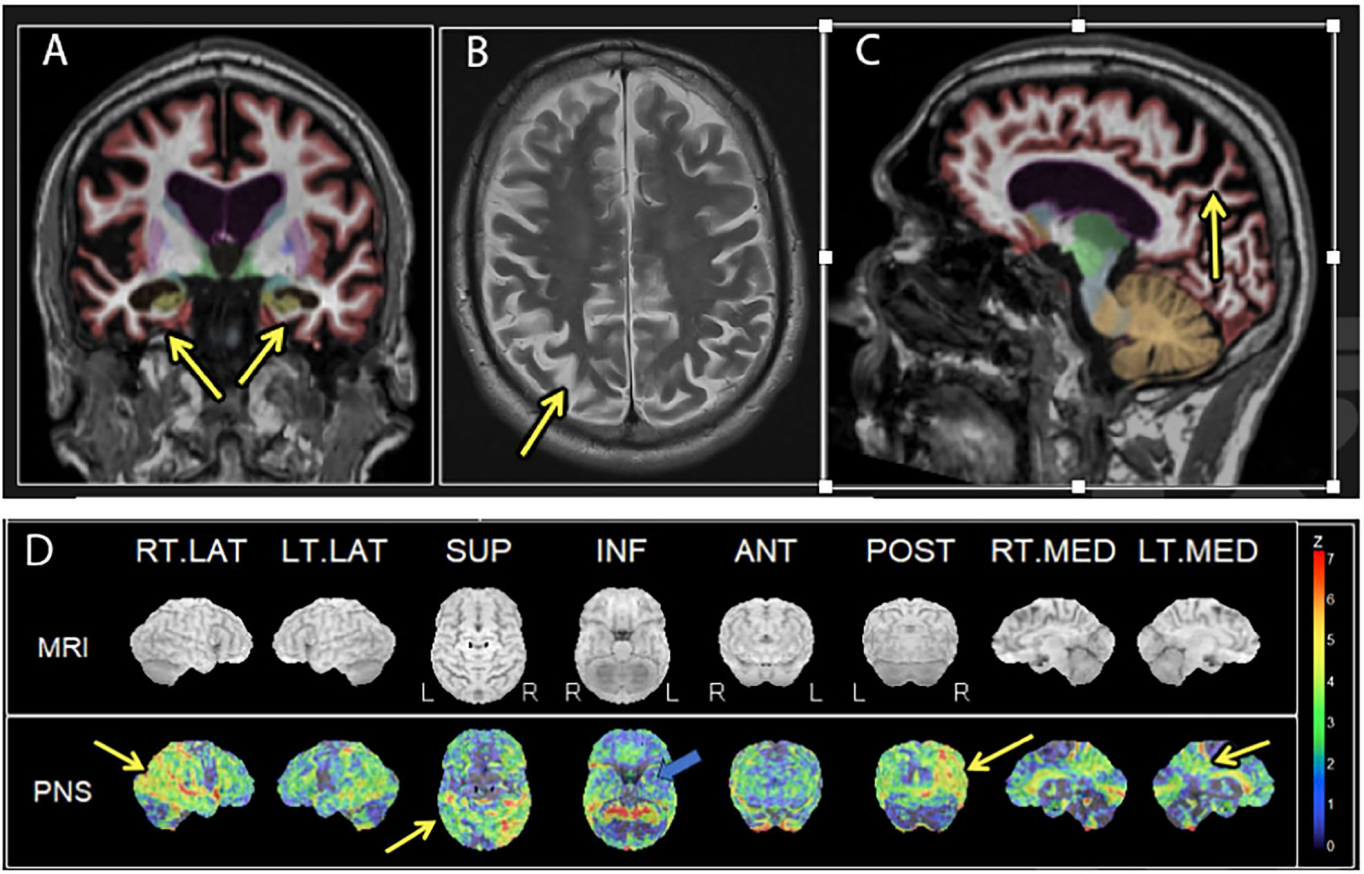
76-year-old male with severe progressive memory loss and cognitive dysfunction. T1-weighted coronal (A), T2-weighted axial (B), and T1-weighted sagittal (C) images of brain MR show parietal and medial temporal volume loss. FDG-PET with 3D-SSP (D) demonstrates profound posterior cingulate, parietal and posterior temporal metabolic reduction, right greater than left (yellow arrows). Hippocampal hypometabolism is mild (blue arrow). The findings are consistent with Alzheimer disease
